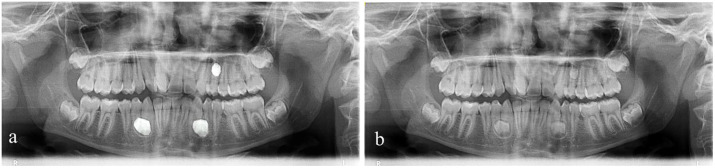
Segmentation of panoramic radiographic. (a) After segmentation, (b) Before segmentation.
